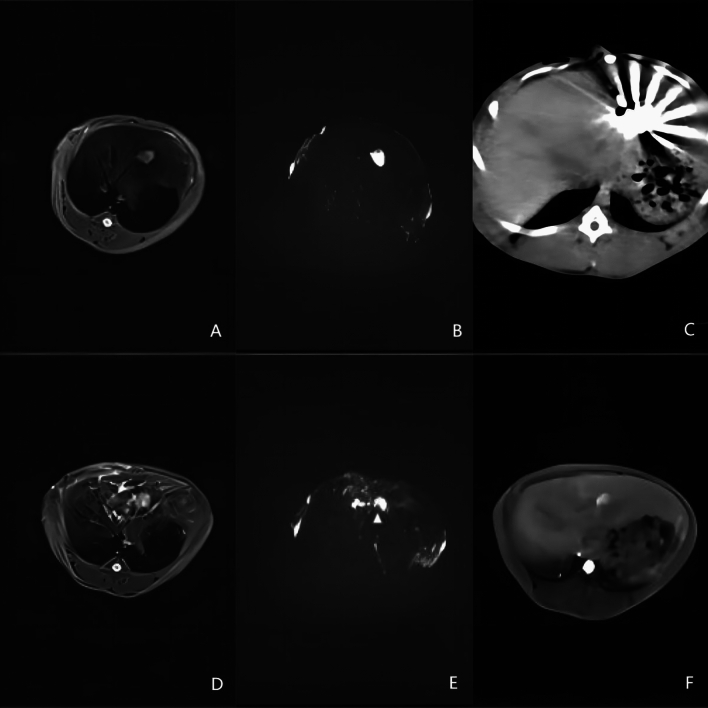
(A) T2WI image of the liver tumor before ablation, with the lesion showing a high signal; (B) DWI image before ablation, the lesion with restricted diffusion showing a high signal; (C) Axial CT image at the time of ablation, with the needle tip placed at the edge of the lesion and gas density visible in the ablation area; (D) T2WI image after ablation, with a decrease in signal in the ablated area and high signal in the residual tumor at its edge; (E) DWI image after ablation, the contour of the high signal area of the lesion can be seen as a defect (arrowhead), suggesting that the tumor is partially ablated; (F) T1WI enhancement image after ablation, residual tumor enhancement can be seen at the ablation margin.
